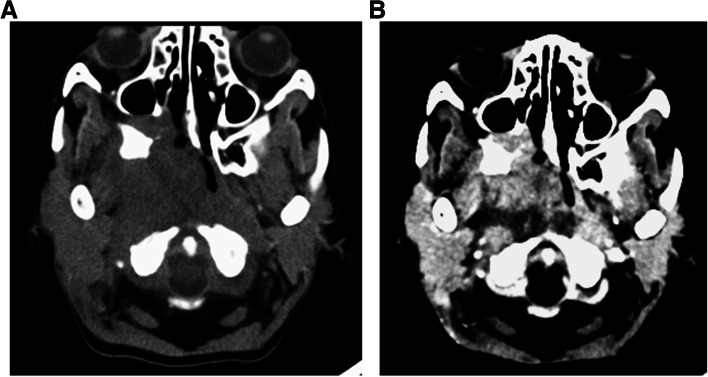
A An unenhanced axial CT at the level of the orbits. There was an iso-dense mass with in the nasopharyngeal space. The mass measured 4.5 cm × 3.4 cm. There were no calcifications seen with in the mass. B A contrast enhanced axial CT at the same level. The mass demonstrated heterogeneous enhancement. This was a diagnosis of nasopharyngeal carcinoma, made on both A and B
